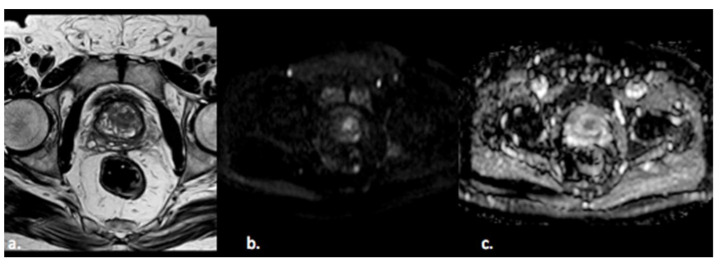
A 55-year old man with a PI-RADS 3 lesion in the left anterior segment of PZ of the midgland, moderately hypointense on T2-weighted images (a), hyperintense on DWI, and hypointense on ADC images (b,c).
